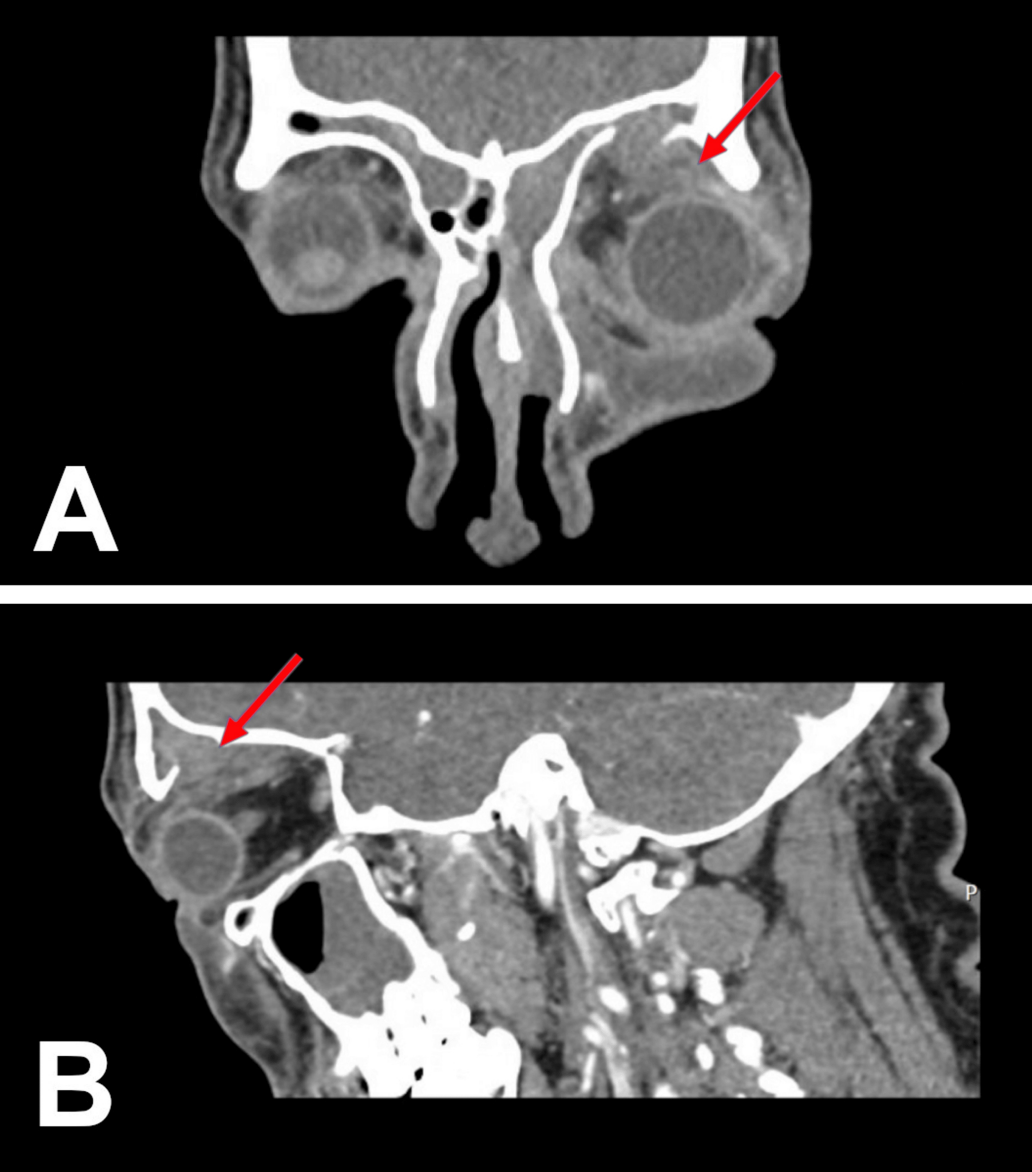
Sagittal and coronal contrast-enhanced CT with red arrows demonstrating the extent of pathology A: Coronal contrast-enhanced CT demonstrating dehiscence of the left frontal sinus floor with inflammatory material extending into the superolateral supraorbital extraconal space (red arrow), forming a subperiosteal abscess. The collection lies lateral to the mid-pupillary line, supporting a lateral transorbital drainage approach. B: Sagittal contrast-enhanced CT demonstrating dehiscence of the left frontal sinus floor (red arrow) with inflammatory material extending inferiorly into the superolateral supraorbital extraconal space, forming a subperiosteal abscess. The collection causes anterior displacement of the globe and correlates with the clinically fluctuant superolateral eyelid swelling.
